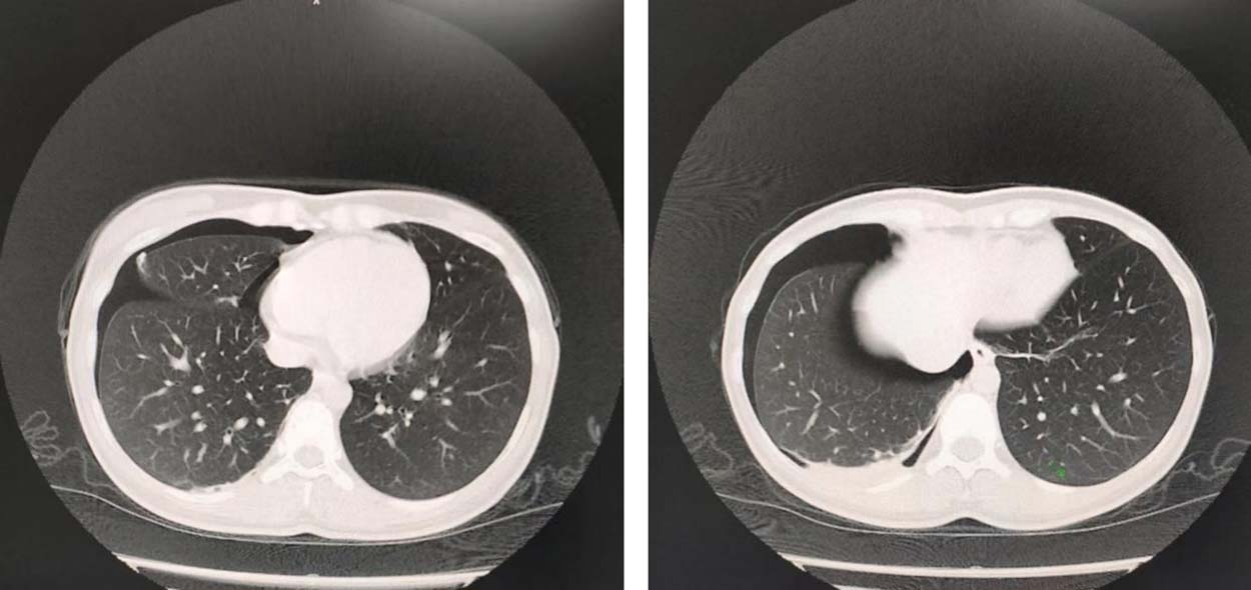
Computed tomography images taken during the patient’s first recurrence after surgery, revealing compressed lung tissue.
